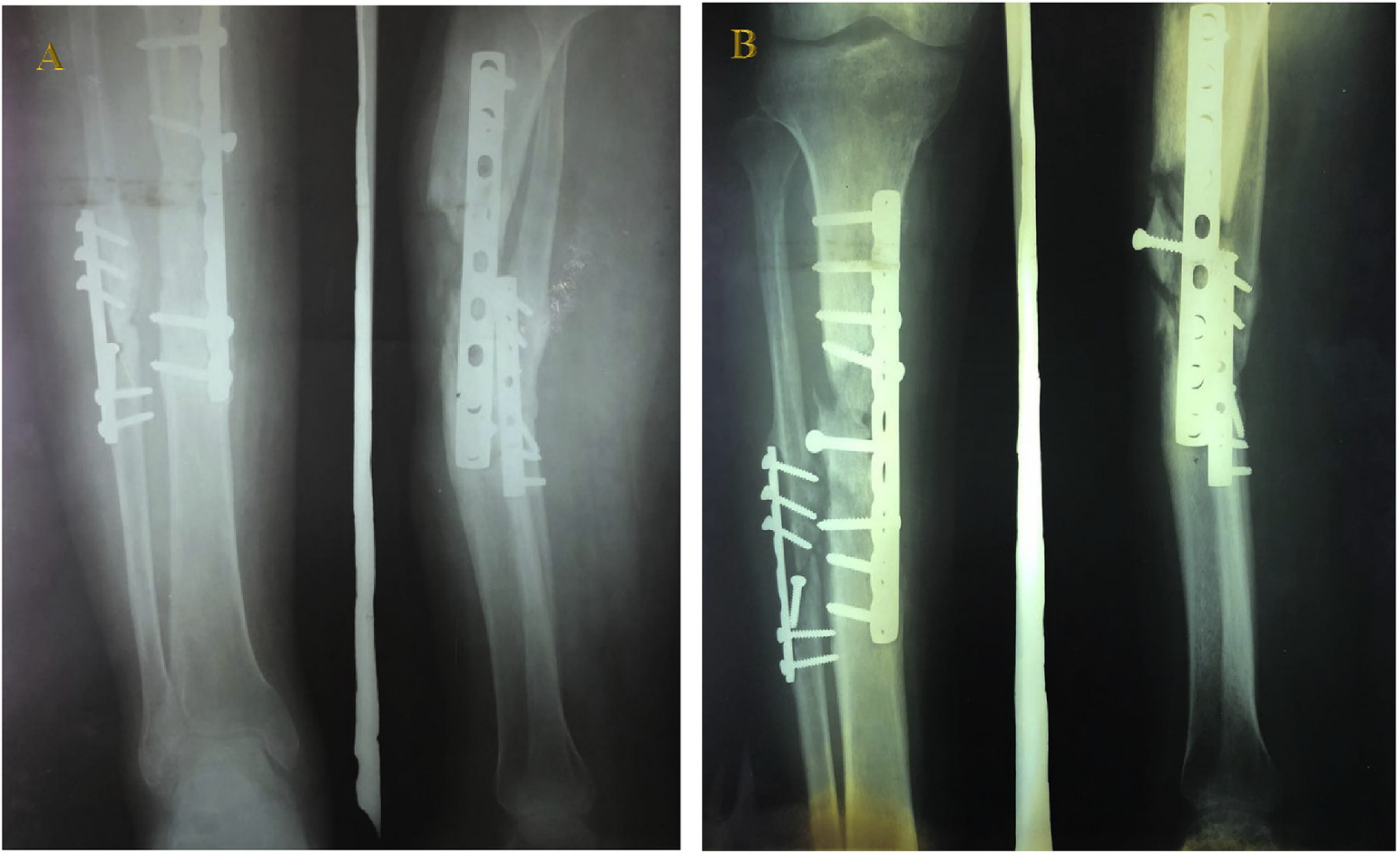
(A) X-ray image of right-leg AP and lateral view showing delayed union and infection of the bone. (B) X-ray image of right-leg AP and lateral view post-debridement and replacement of internal fixation.
